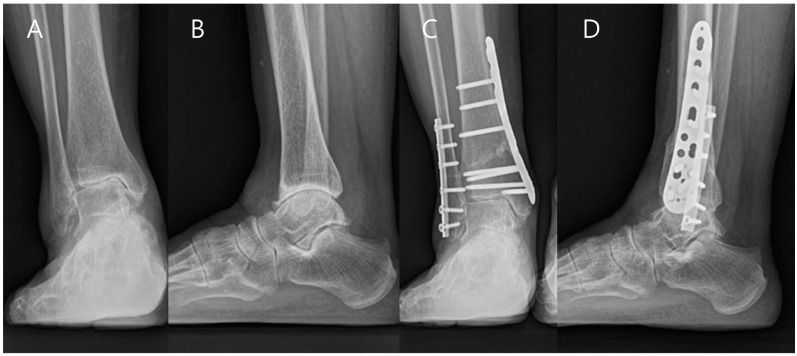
Preoperative radiographs showing stage 3B arthritis with talar tilt (A,B). Radiographs taken 2 years after supramalleolar osteotomy combined with posterior tibial tendon release showing stage 2 arthritis with improvement of talar tilt (C,D).
